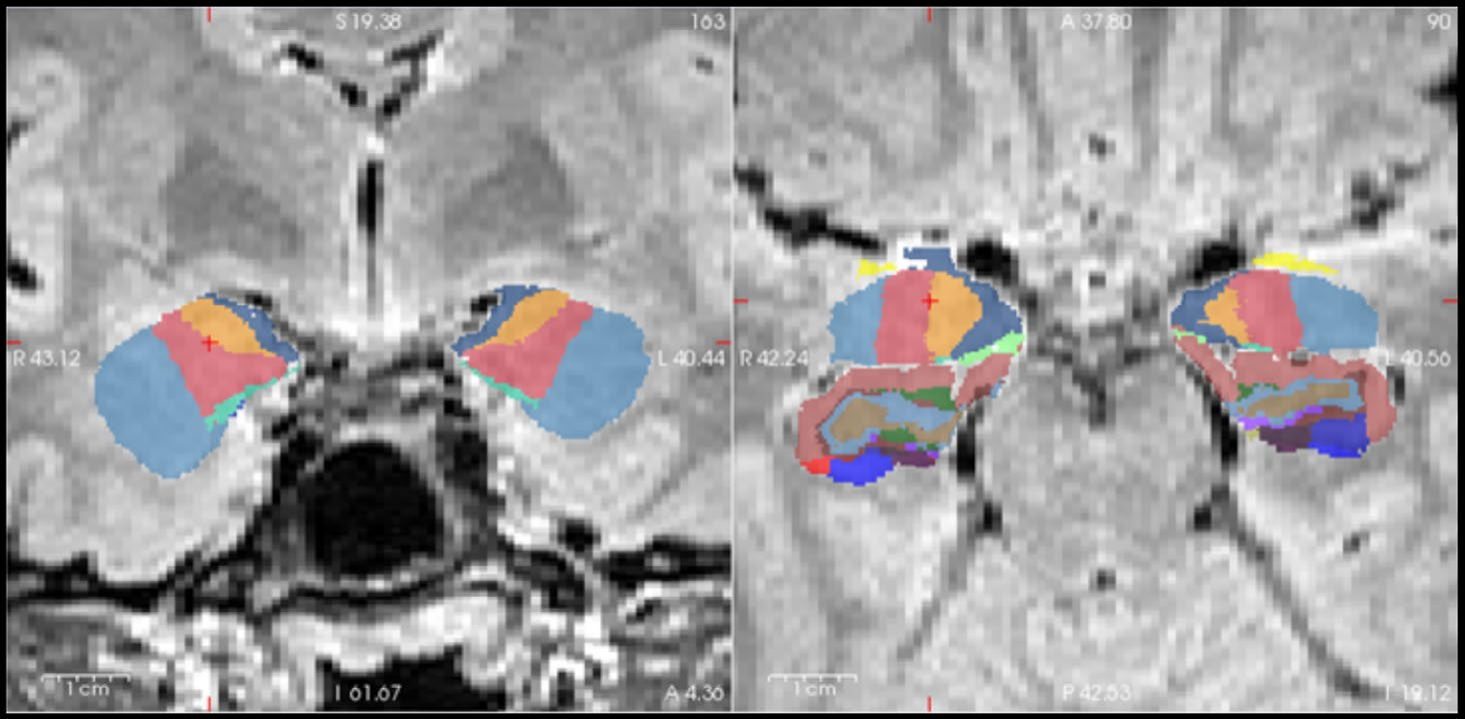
Example of right‐sided amygdala enlargement on automatic segmentation. Exemplary right‐sided amygdala enlargement after multiparametric segmentation. Compared to the healthy control cohort, only the right amygdala was enlarged (*z*‐score = 4.8). The amygdala nuclei are shown in blue/red/orange, and the amygdala‐hippocampal transition zone is shown in light green. The hippocampi are also visualized (coronal and axial T2‐weighted FLAIR sections); FLAIR, fluid‐attenuated inversion recovery.
